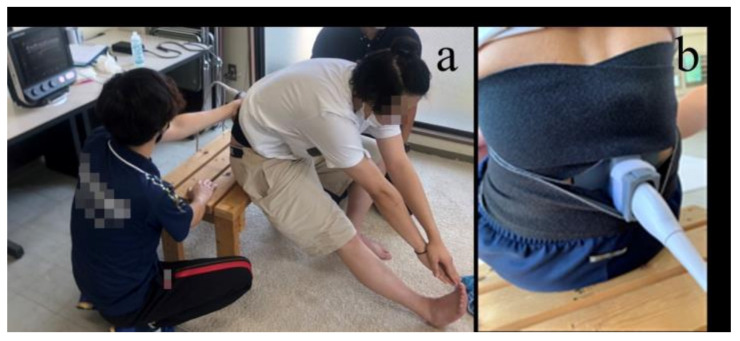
The movement of the myofascial membrane of the lower back is imaged with an ultrasonic diagnostic imaging device. (a) The movement of the lower back when the trunk bends forward and returns is measured; (b) the probe is attached to the low back with a fixation device created with a 3D printer.
